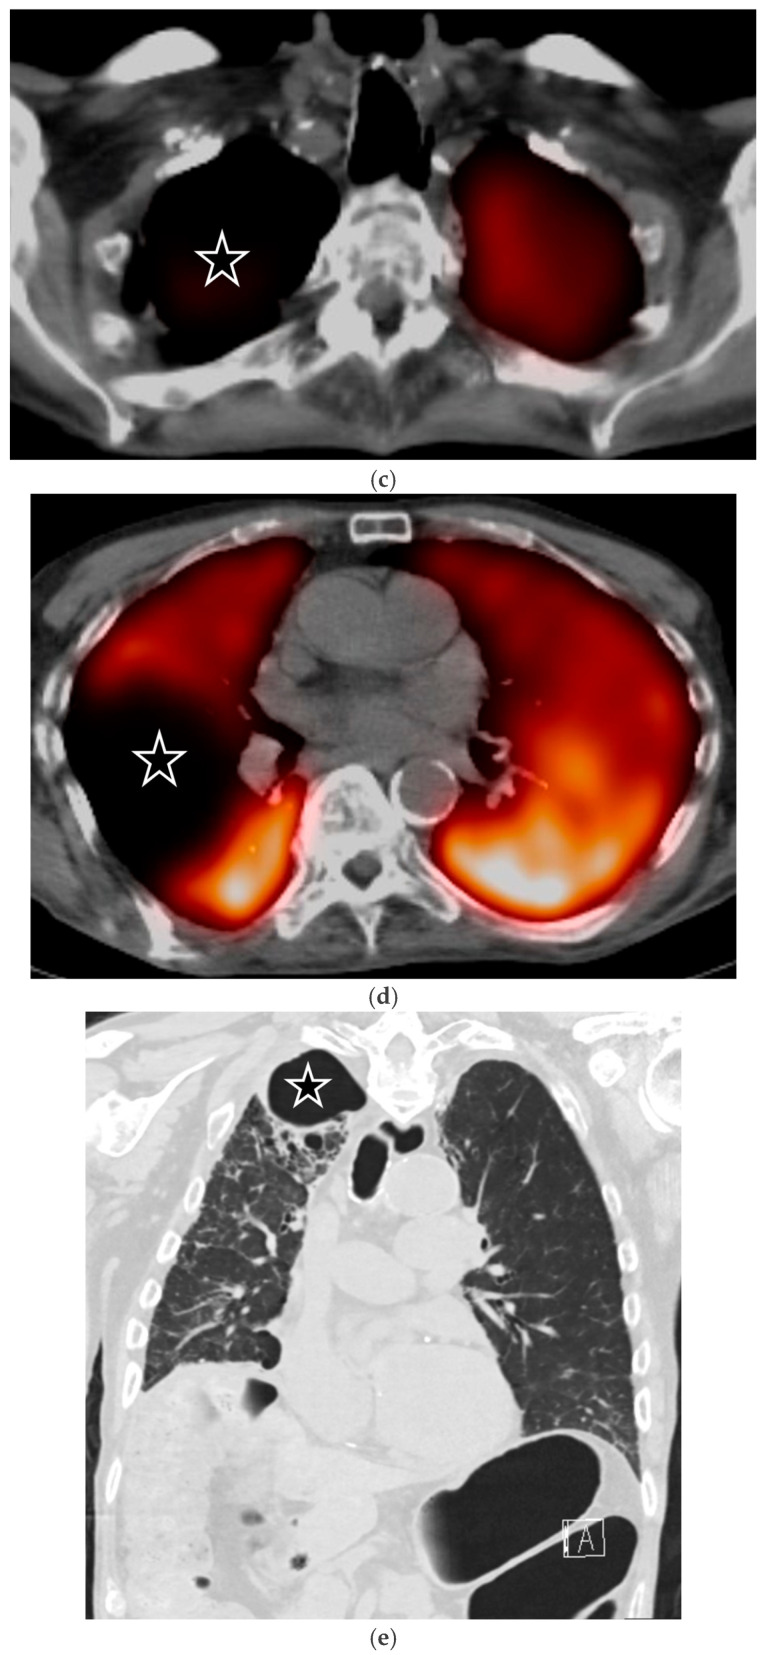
72-year-old male with bullous emphysema presents with dyspnea. Axial and sagittal CT images in lung window (a,b) demonstrate right apical bulla (black star) and loculated right pneumothorax (white star). Axial perfusion SPECT/CT images (c,d) reveal no radiotracer uptake in the right apical bleb or loculated right pneumothorax (black star). Subsequent coronal CT image in lung window (e) shows interval resolution of loculated pneumothorax after insertion of chest tube with persistence of right apical bulla (black star).
